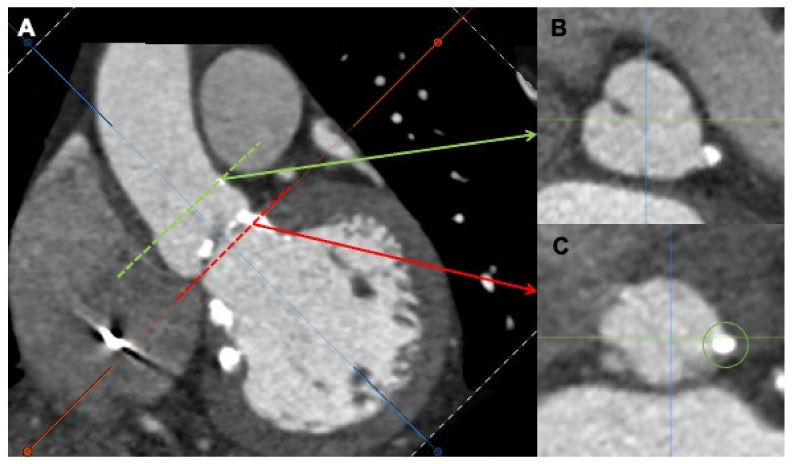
Multidetector computed tomography angiography (MDCTA) showing severe calcification at the level of the left coronary cusp (A). Transversal views at the left coronary artery (B), green arrow and the aortic ring (C), red arrow.
